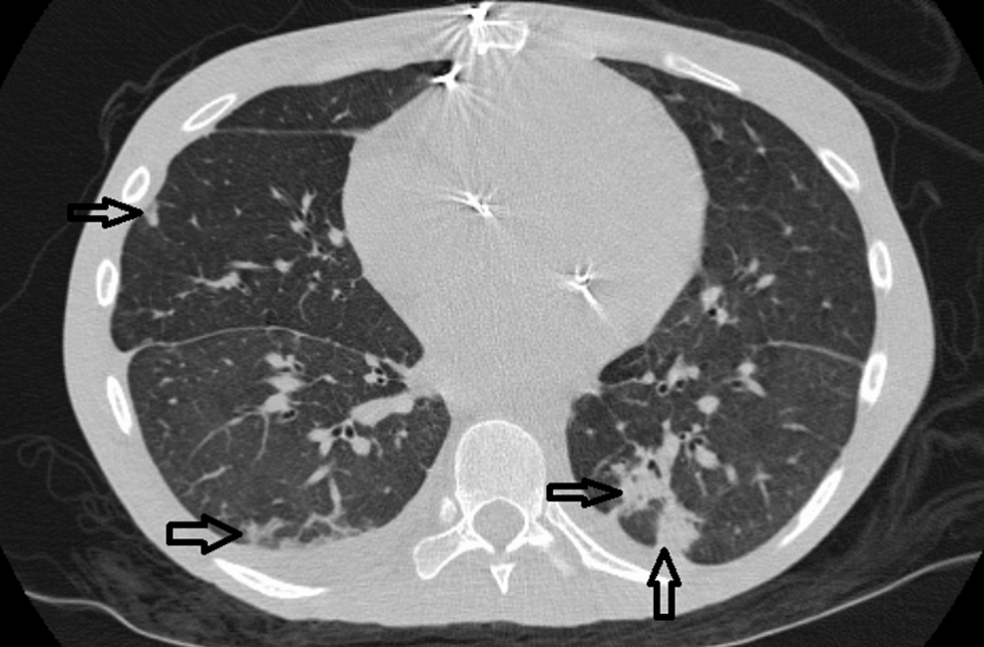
CT chest without contrast: Arrows indicate suspected peripheral wedge-shaped infarcts from endocarditis.
